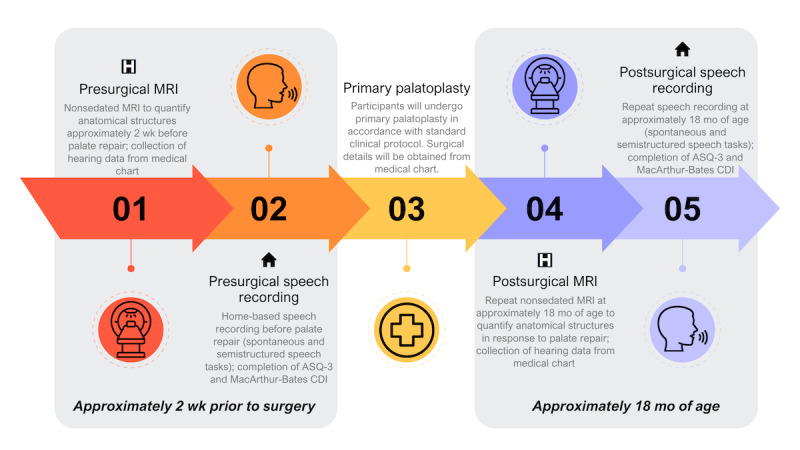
Participant-level timeline for multimodal data collection throughout the study. ASQ-3: Ages and Stages Questionnaire, Third Edition; CDI: Communicative Development Inventories; MRI: magnetic resonance imaging.
